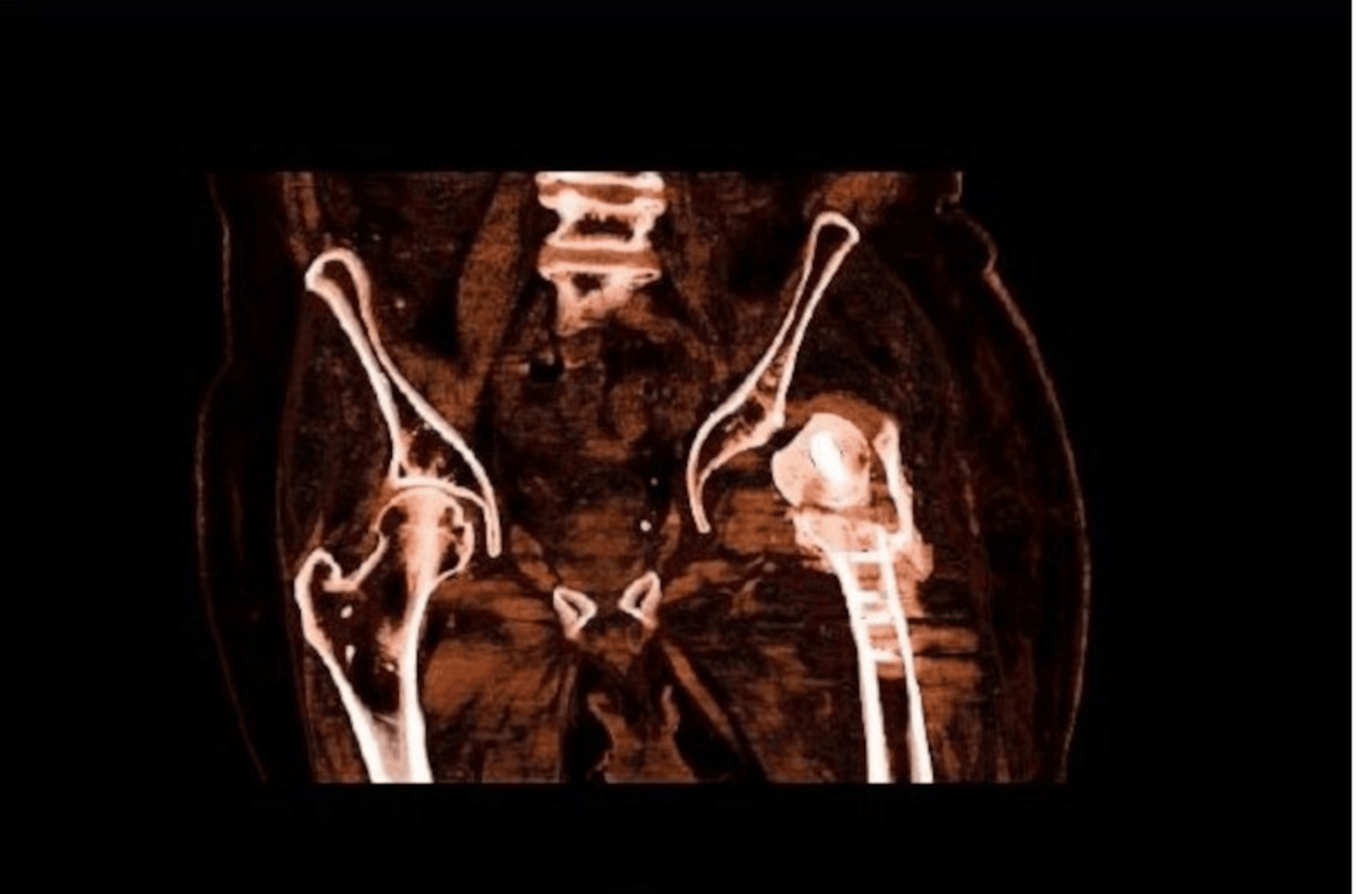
CT pelvic coronal view scan The image shows the left non-united pertrochanteic fracture, dislocated left hip, left acetabulum wall, and femoral head fracture CT: computed tomography
